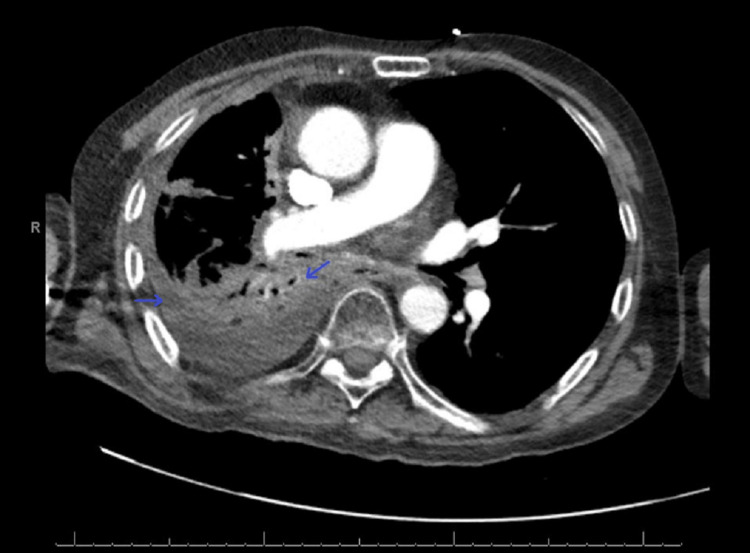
CTA chest with contrast Arrow: pneumonia superimposed on an extensive tumor burden CTA: computed tomography angiography
